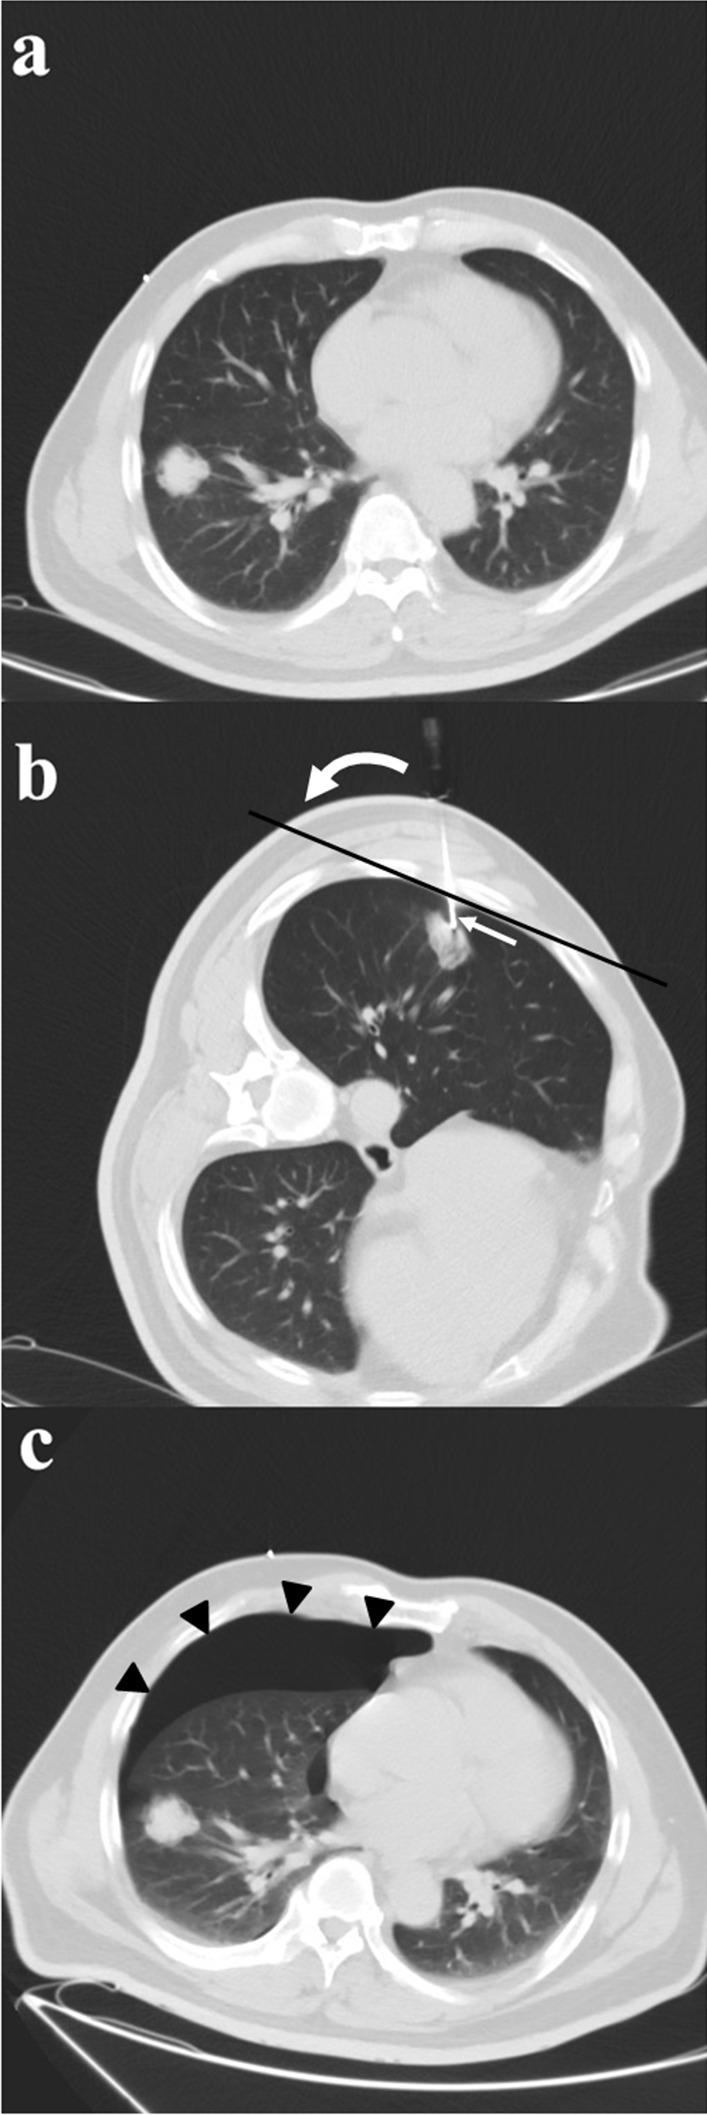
a CT-guided core needle biopsy of a solitary suspected lesion in the right lower lobe, in a 56-year-old male patient with 6 pack-years smoking. b Showed the biopsy needle (arrow) inserted within the lesion, which was later pathologically confirmed to be pulmonary adenocarcinoma. The patient was in lateral position on the CT table. The needle-pleural angle (curved white arrow), which was the minimum angle formed by a line tangent to the pleura at the puncture point and a line drawn along the needle, was 63°. The length of the needle track from the pleura to the lesion was 14.4 mm. The dwell time was 660 s. c CT image after the removal of the biopsy needle showed pneumothorax (arrowhead), which continued to increase until the chest drainage catheter was inserted
